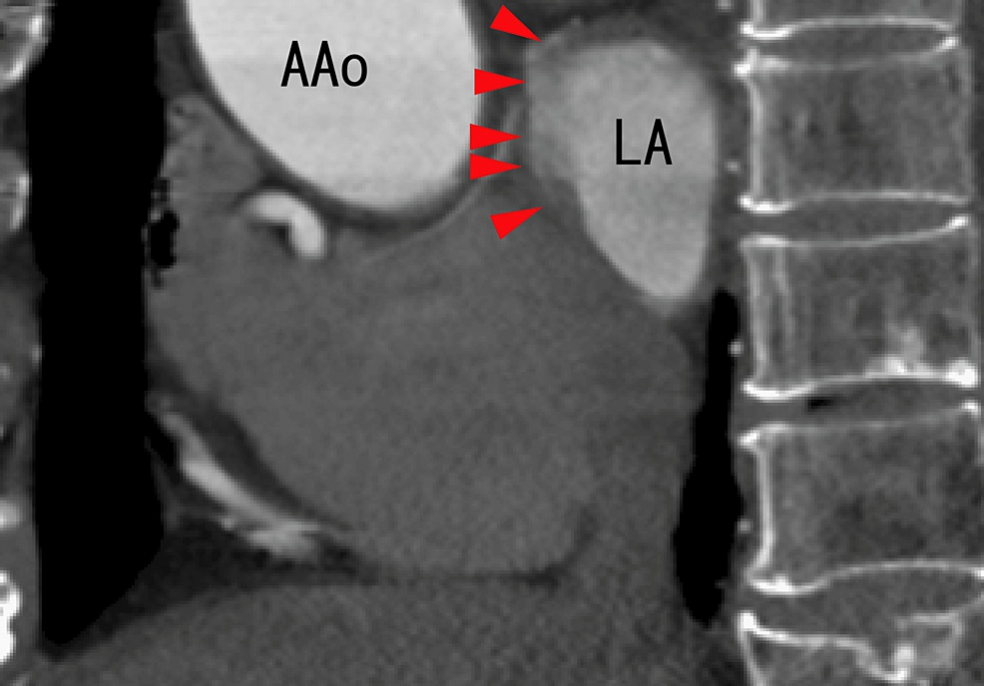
Sagittal images from an 80-MDCT scan revealing the LA and LAD There were more dark areas than LAs (arrowheads) on the anterior wall of the LA, suggesting that the diverticula were full of thrombi. The images of thrombi on the upper side of the LA are shown as darker areas. AAo, ascending aorta; LA, left atrium; MDCT, multidetector computed tomography
